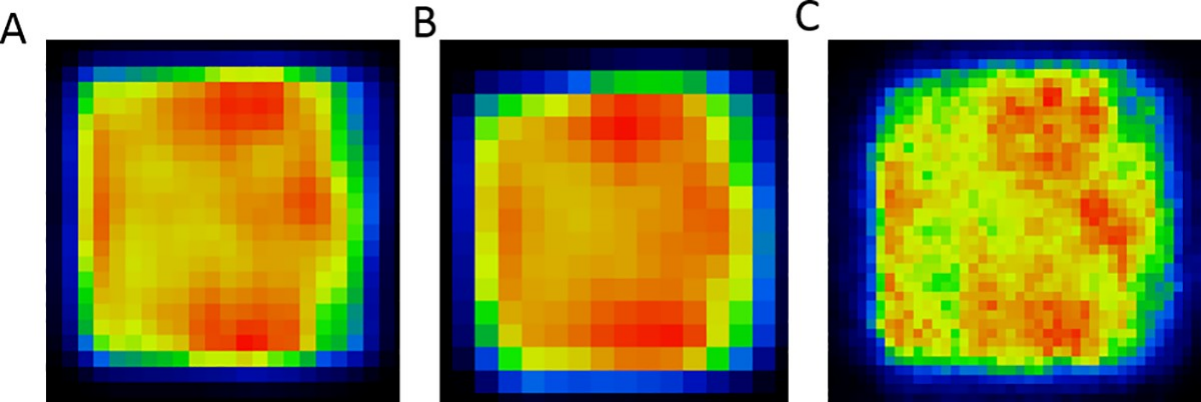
Representative transaxial slices of the same imitated lesion: measured and reconstructed by GE Discovery MI PET/CT (A), Mediso AnyScan PET/CT (B), Mediso nanoScan PET/MRI (C).
